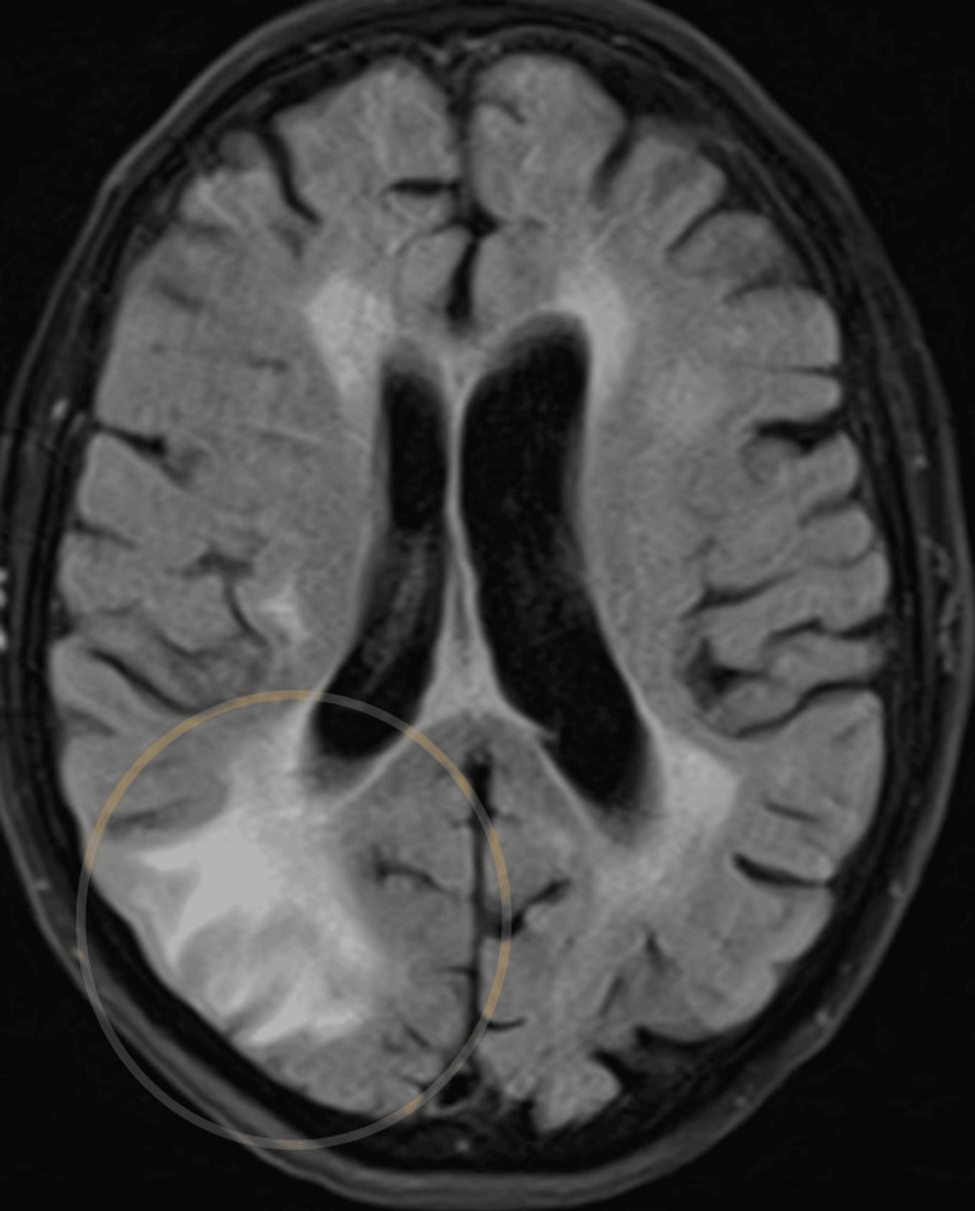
Repeat MRI brain on day 15 of admission: cerebral section MRI brain demonstrates ongoing abnormality in the right parieto-occipital region with evidence of evolution. Surrounding vasogenic edema is again noted, with mild increased extent of the edema medially compared to the previous MRI. The lesion is demarcated by a yellow circle.
